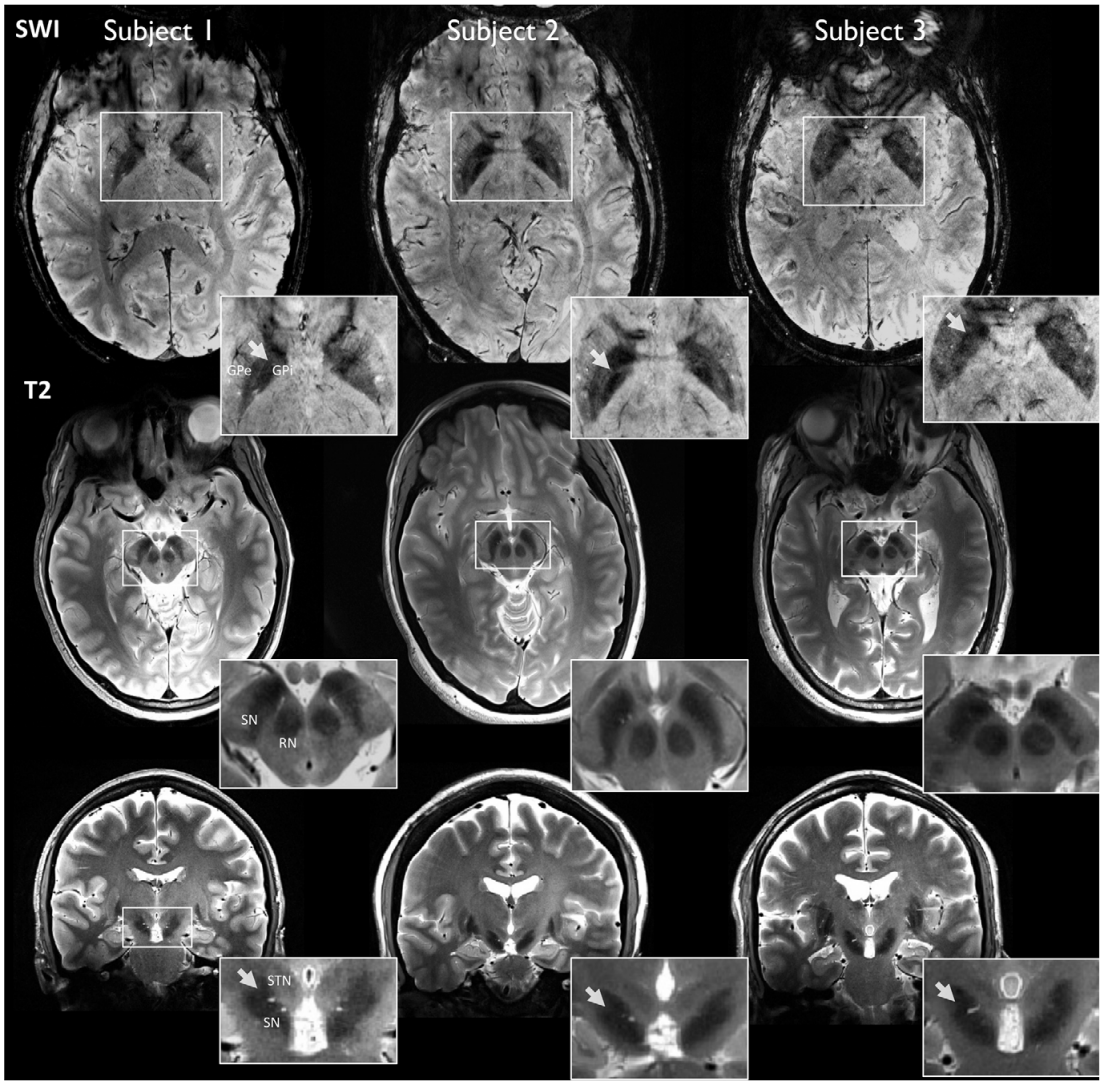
Structural imaging at 7T. (Top row) Axial high-resolution susceptibility-weighted images (SWI) in three subjects, at the level of the globus pallidus (GP), putamen and thalamus. 7T SWI provides high contrast between structures such as the external (GPe) and internal (GPi) segments of GP, as can be seen in each inset in the uppermost row. The white arrows indicate the border between GPe and GPi, known as the *lamina pallidi medialis* (uppermost insets). Axial high-resolution T2-weighted images in three subjects at the level of the substantia nigra (SN) and red nucleus (RN) are presented in the middle row. Coronal T2-weighted images in three subjects at the level of the subthalamic nucleus (STN) and SN (bottom row). Coronal images provide good contrast enabling differentiation between SN and STN along the lateral-medial axis, as indicated by the white arrow in each inset. SWI and T2-weighted images were co-registered and both used to segment GPe, GPi, SN, and STN. More details on the advantages of 7T SWI and T2-weighted imaging can be found in [33].
